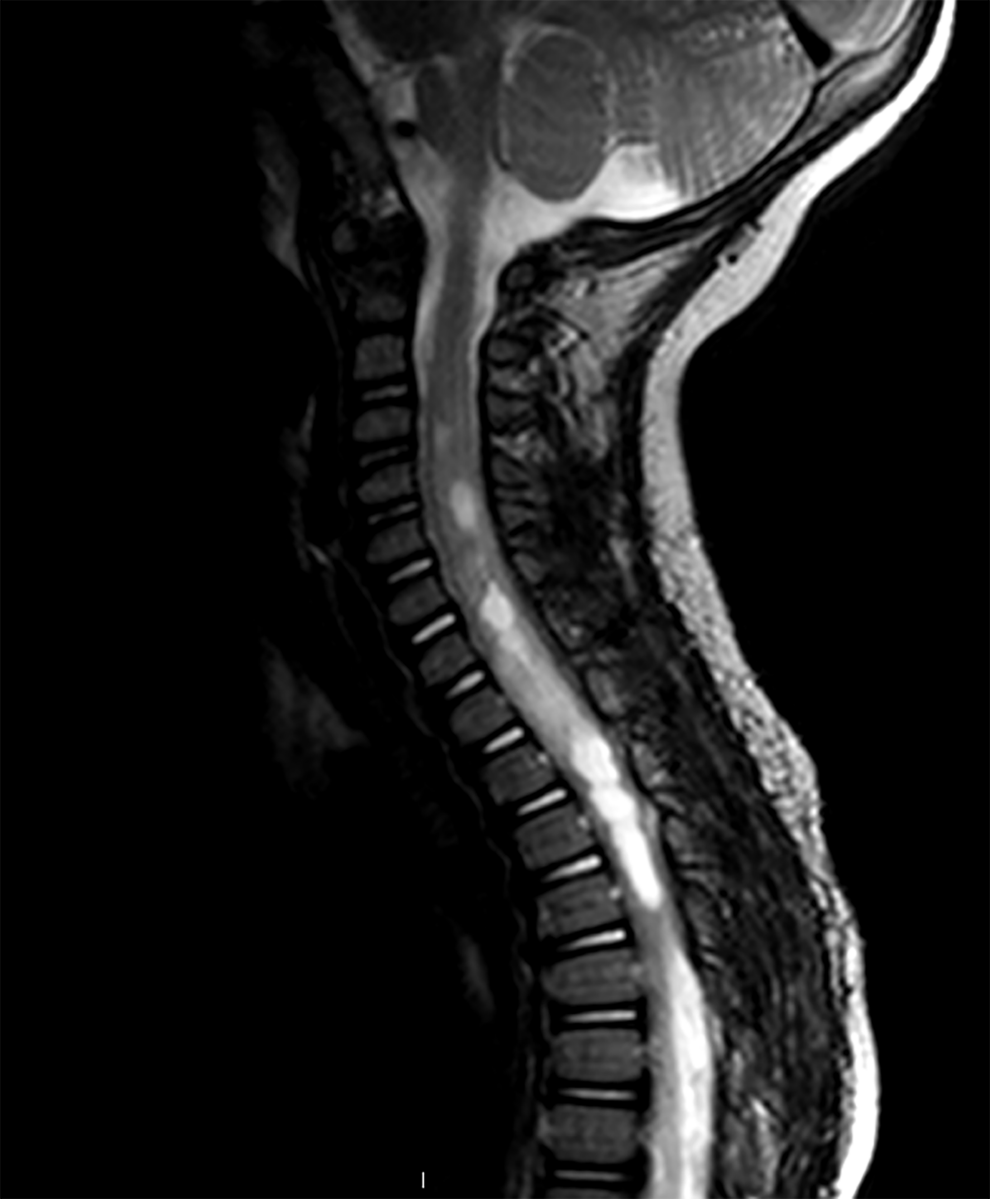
Sagittal T2-weighted MRI of pediatric patient with an intramedullary mass extending from C4-T3. Increased T2 signal is seen both cranially and caudally to the tumor. The lesion appears enhancing, although several areas of central non-enhancement consistent with necrosis are visible. Pathology was consistent with a Grade 2 astrocytoma.
